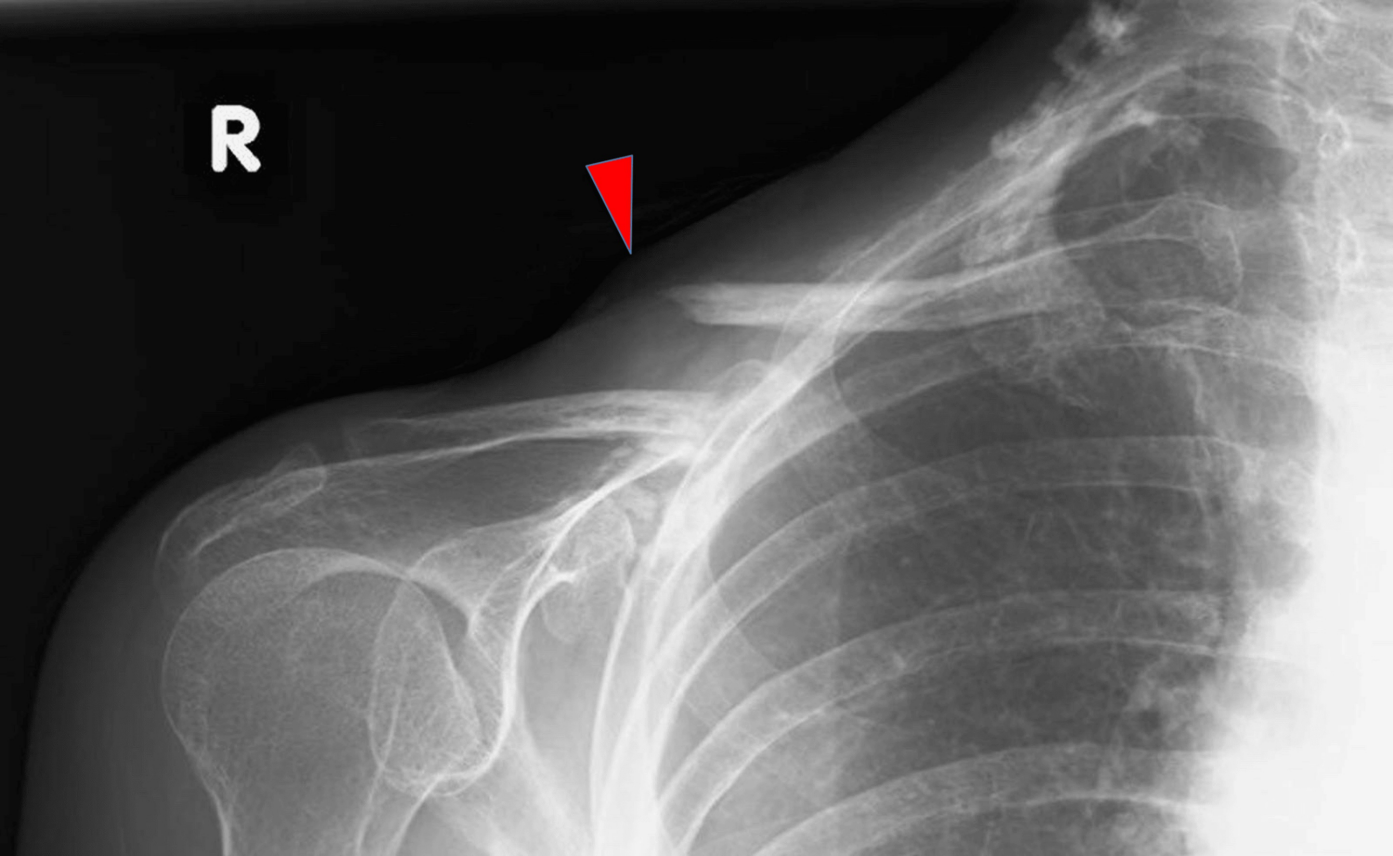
Radiograph of the right shoulder. Arrow indicates the site of the fracture.
